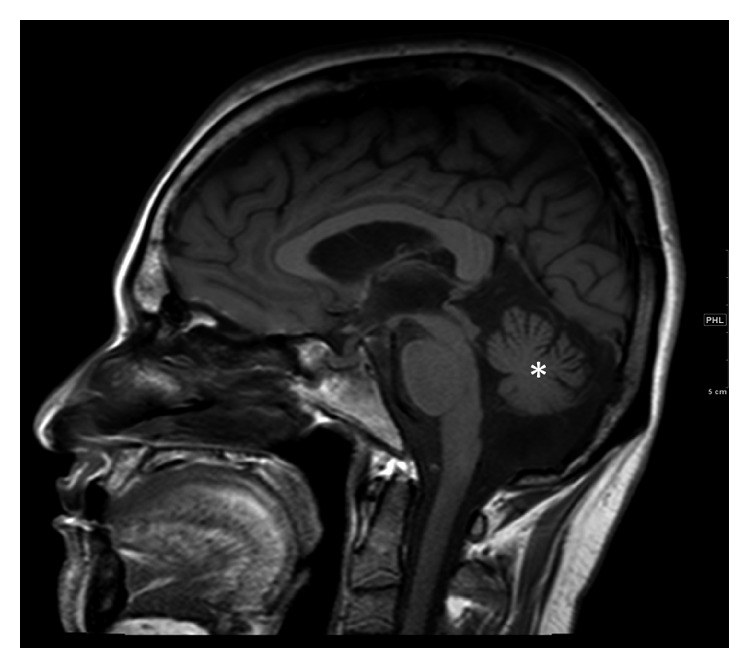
T1-weighted sagittal MRI of the brain shows uniform cerebellar loss.
